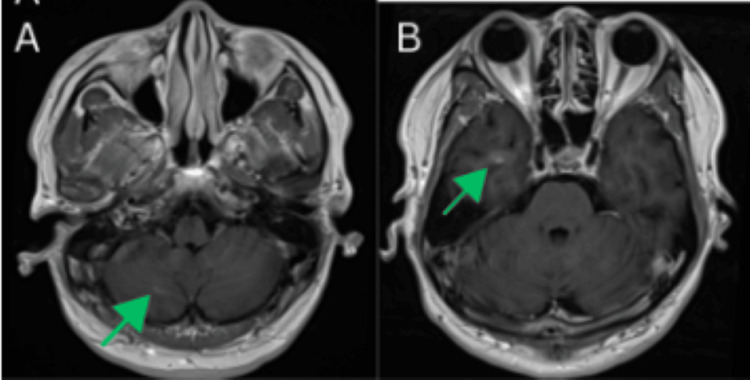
Brain magnetic resonance imaging (MRI) performed at the five-month follow-up. Follow-up magnetic resonance imaging (MRI) demonstrates small enhancing intra-axial lesions in the right inferomedial cerebellum (A) and the right inferior temporal lobe (B), both of which appear stable with no significant change in size, enhancement pattern, or surrounding edema compared to prior studies. These findings suggest no radiographic evidence of progression at this time.
